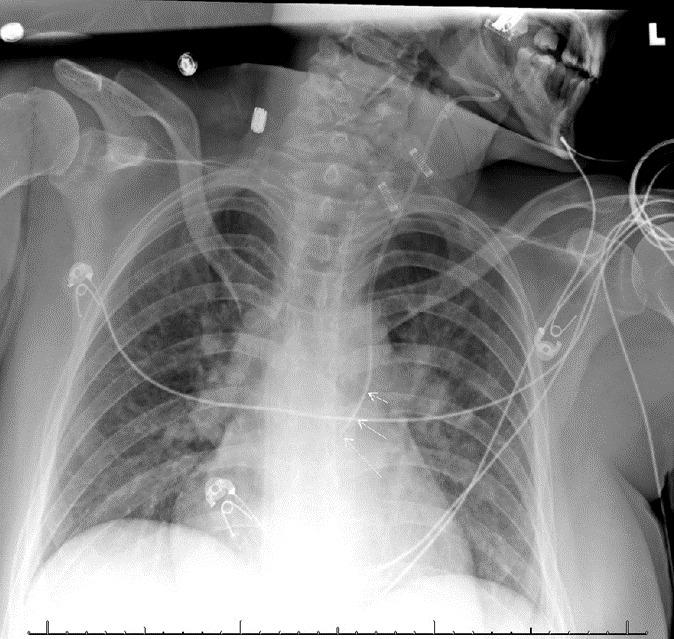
Anterior-posterior chest radiograph demonstrating the central venous catheter coursing atypically, over the left mediastinum, not crossing the midline (arrows).
